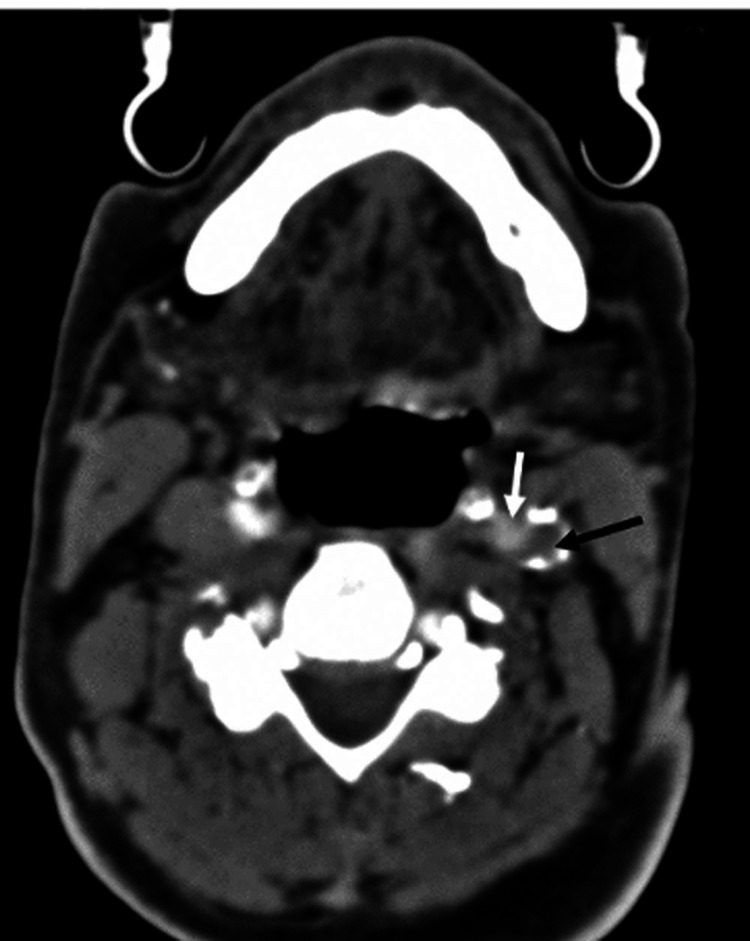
Axial computed tomography of the carotids Axial computed tomography of the carotid revealed opacification and almost complete occlusion of both the external carotid represented by the white arrow, the internal carotid by the black arrow.
